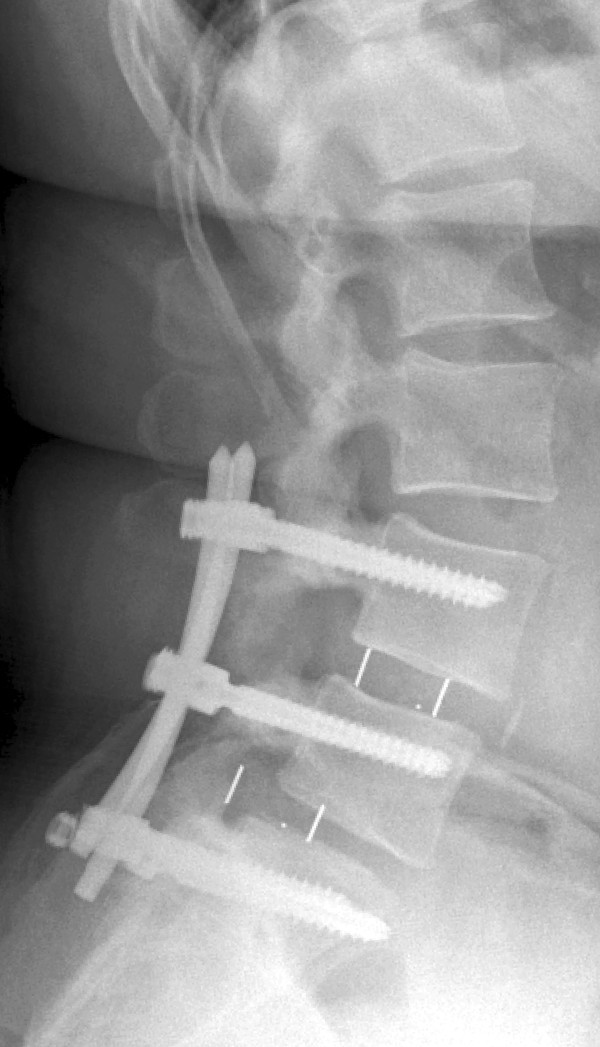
X-ray taken at first follow-up examination.
